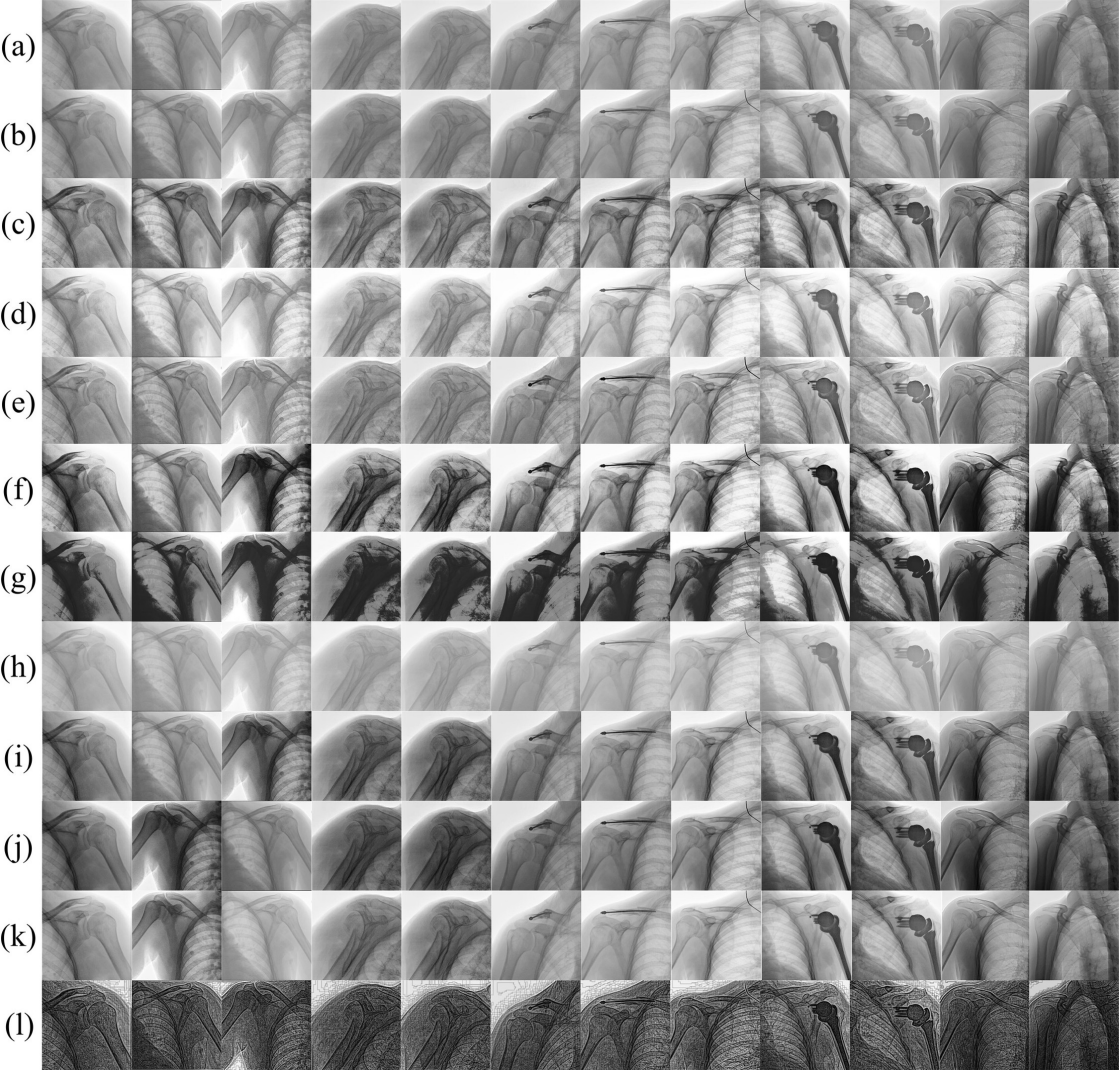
Comparison of X-ray enhancement results between the proposed method and 10 other traditional X-ray enhancement techniques. The images are arranged from left to right, with Image 1 to Image 10 representing the results of the respective traditional enhancement techniques. (a) Input, (b) CECI [39], (c) CLAHE [40], (d) ECE [41], (e) EGIF [42], (f) FCCE [43], (g) FCE [44], (h) GC [45], (i) HLIPSCS [46], (j) LCA [47], (k) RCEA [48], and (l) proposed method.
